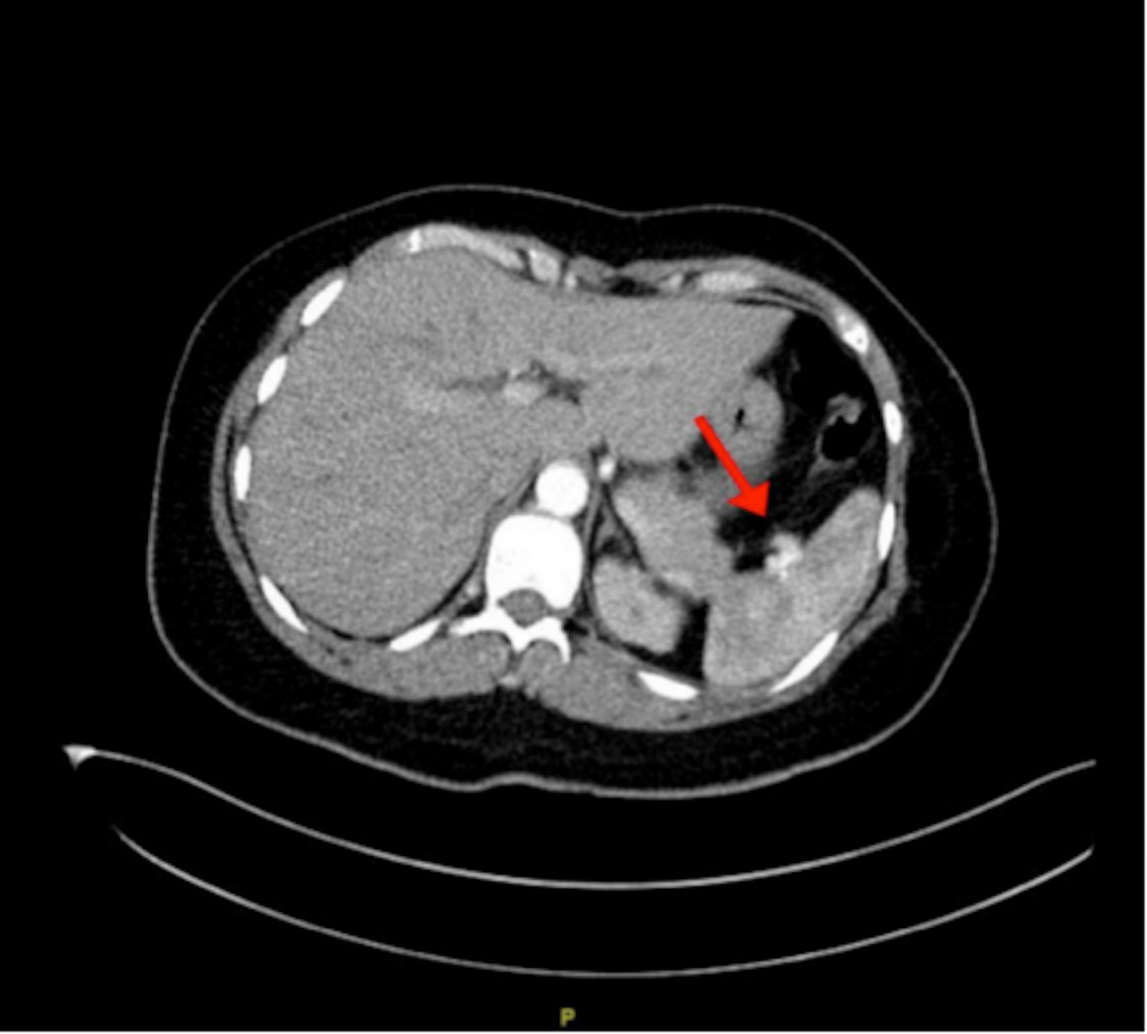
CT scan revealing an unruptured hilar splenic artery aneurysm
